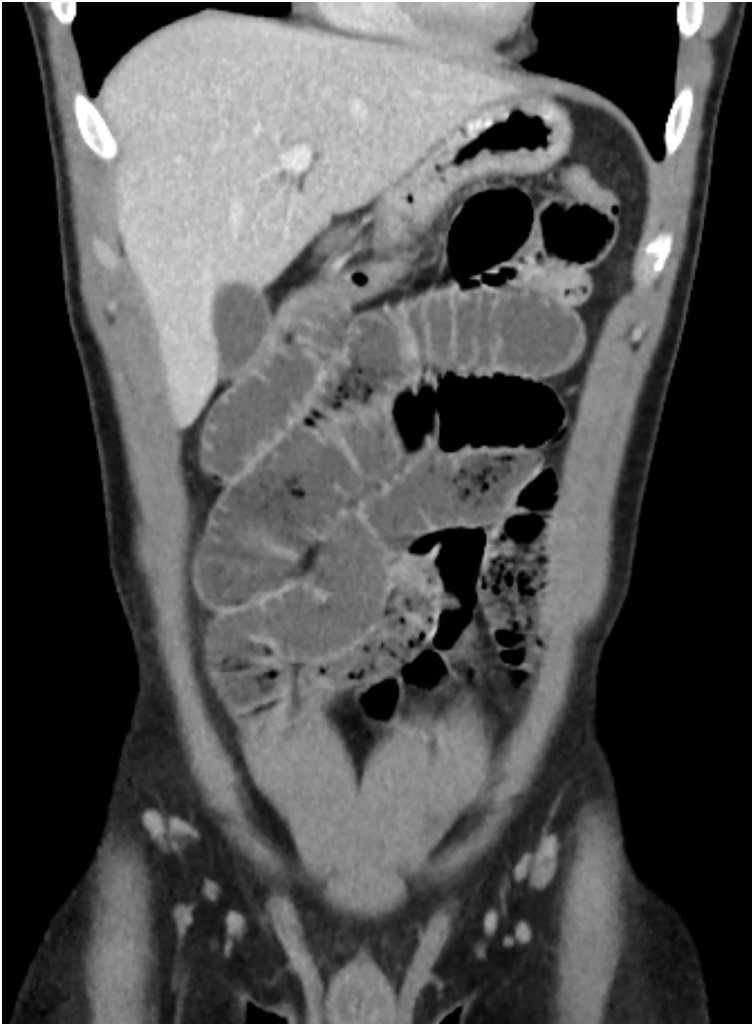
Coronal view of CT abdomen and pelvis on admission*.*
